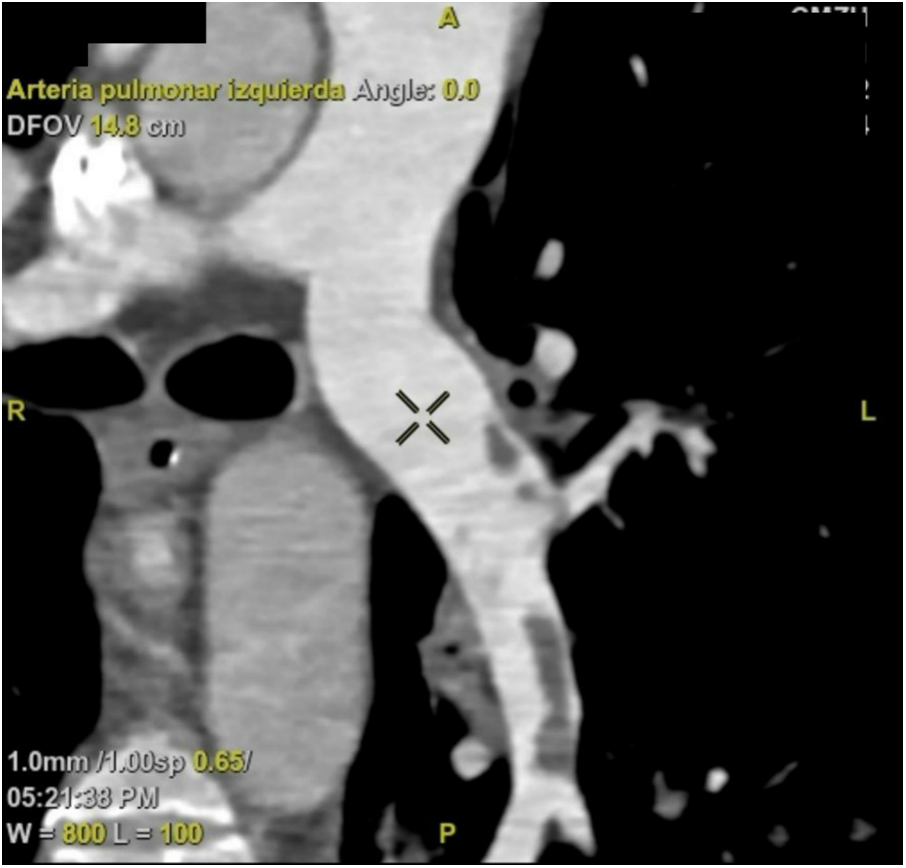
CT angiography. The left pulmonary artery exhibits a partial filling defect in the segmental and subsegmental branches. CT, computed tomography.
